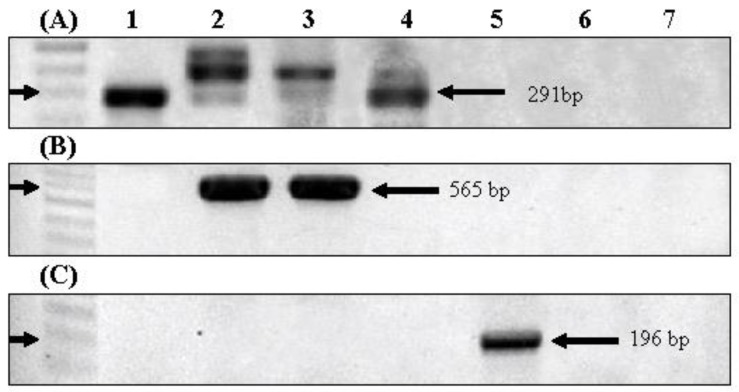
Agarose gel electrophoresis of PCR amplicons for (A) microcystin (*mcyA* gene), (B) geosmin (*gys* gene), and (C) 2-MIB (*mibC* gene) synthesis genes. 1: *Microcystis aeruginosa*, 2: *Dolichospermum circinale* (*Anabaena circinalis*) ST, 3: *D. circinale* (*A. circinalis*) CT, 4: *Aphanizomenon flos-aquae*, 5: *Oscillatoria limosa*, 6: *Planktothricoides raciborskii*, 7: *Pseudanabaena mucicola*.
